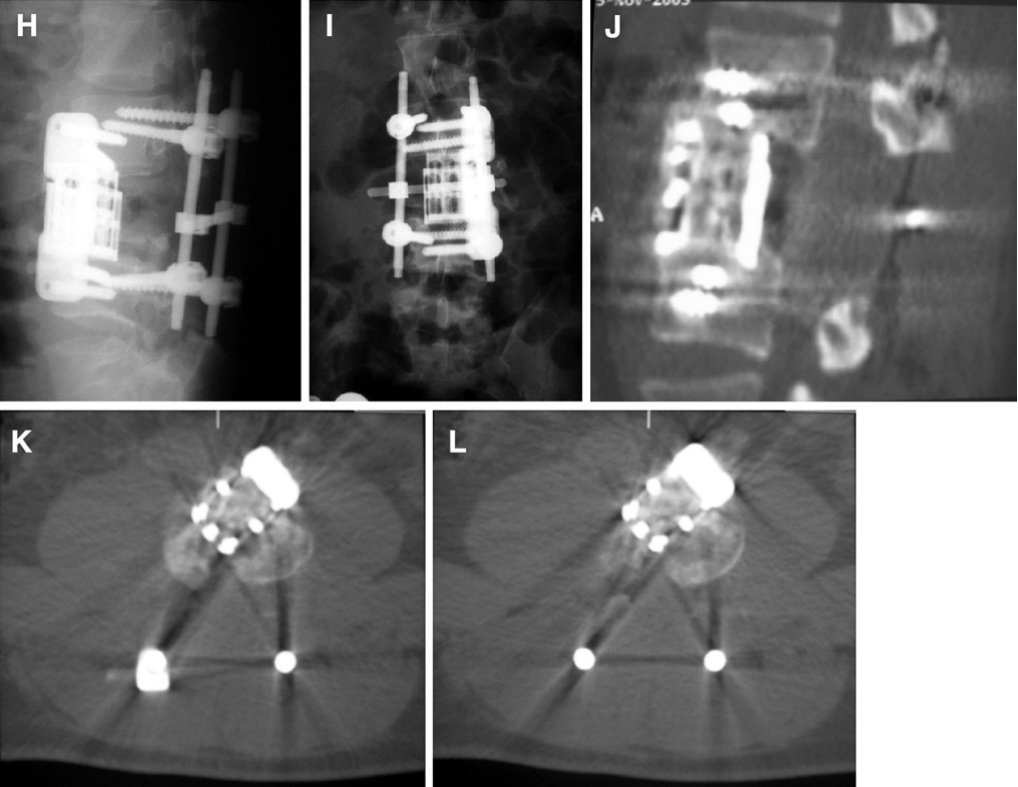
a–l Case # 7. A 30-year-old male was admitted to our department with low-back and leg pain for 2 years. There were no neurological deficits. MR and CT images revealed an L3 aneurysmal bone cyst with moderate canal compromise. There were fluid–fluid levels, but no soft tissue mass. WBB scale was 4–10 ABCD. A combined surgical approach (first anterior, then posterior) with gross total removal was performed. A vertebral body cage and posterior pedicle fixation system were used to reconstruct and stabilize the spine. There was no recurrence during the 26-month follow-up time
